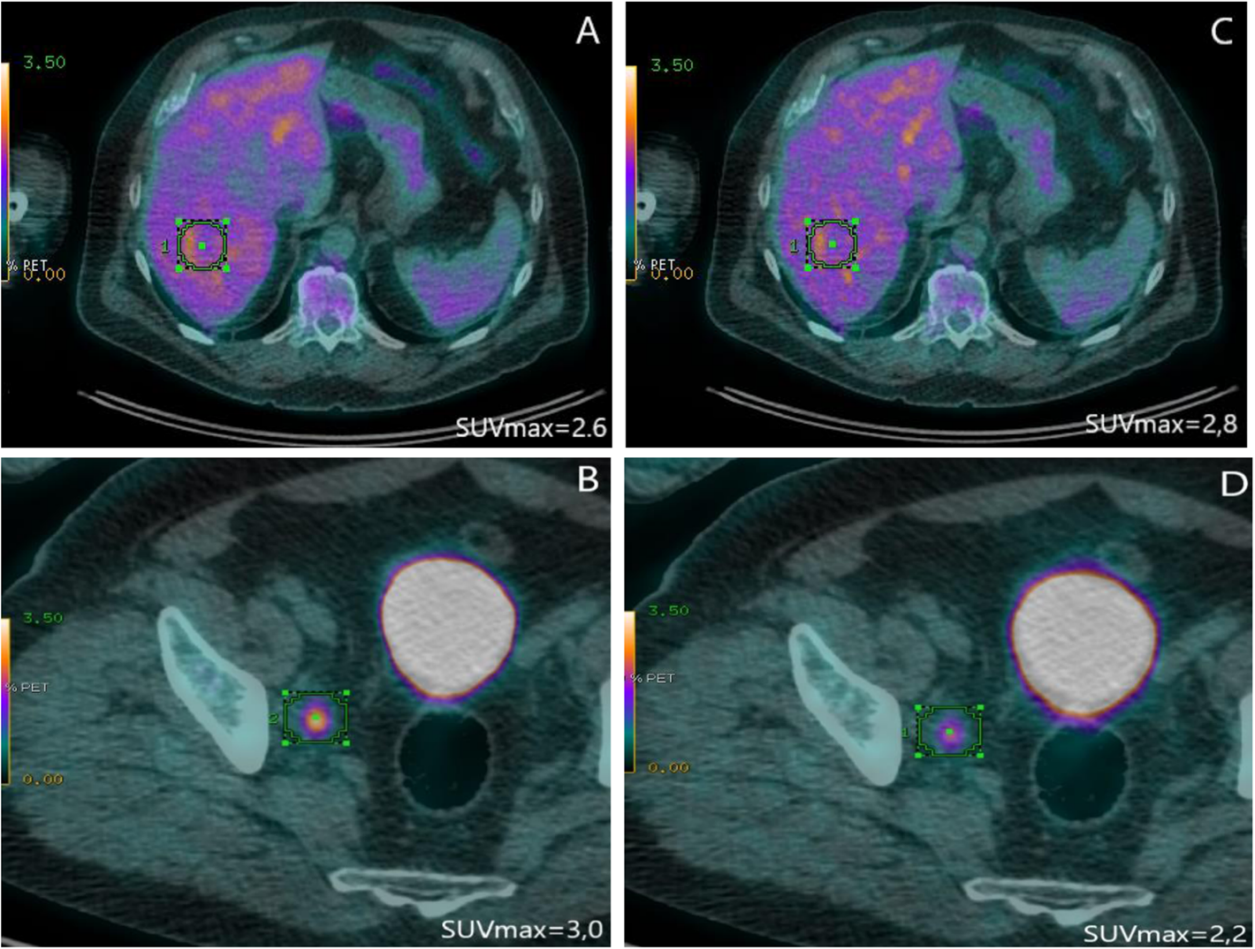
SUVmax values in the liver (a) and in the target lesion (b) using Q.Clear, and liver (c) and the target lesion (d) using OSEM
